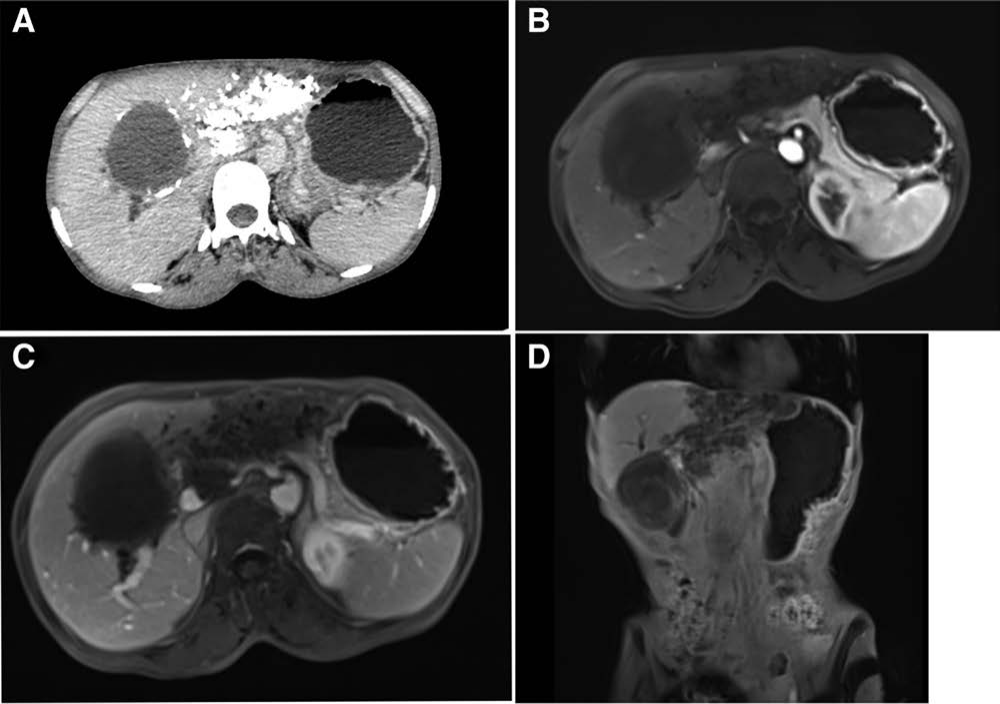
CT and MRI imaging of the lesions: preoperative abdominal CT and MRI of patients showed that there were two lesions of echinococcosis, one in the liver with the size of about 6.7 cm × 10.9 cm in S2–4 segments, and the other with the size of about 7.9 cm × 7.3 cm in S5 segment.
